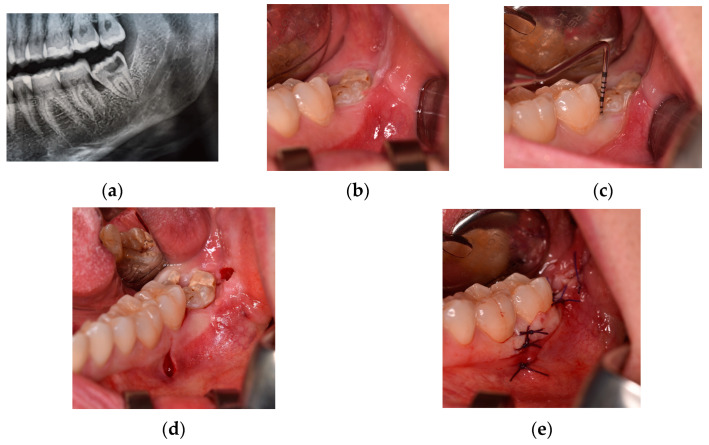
Trapezoidal flap (class IB position): (a) pre-operative detail of panoramic radiograph; (b) pre-operative clinical evaluation; (c) pre-operative periodontal probing of pocket at distal site of the second molar; (d) intra-operative mesial- and distal-releasing incisions; and (e) final wound closure.
